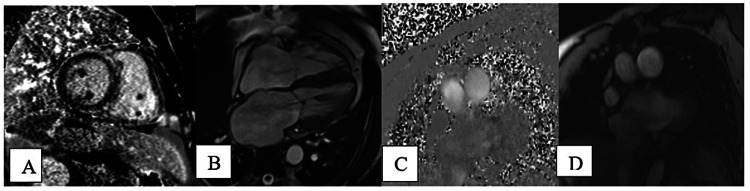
Cardiac MRI with post gadolinium and phase contrast images. A: Post-gadolinium short axis image shows RV insertion site enhancement. B: Four-chamber SSFP image shows right ventricular dilatation and thickening of the right ventricular wall. C and D: Phase and magnitude images at the level of the prestenotic pulmonary artery.
